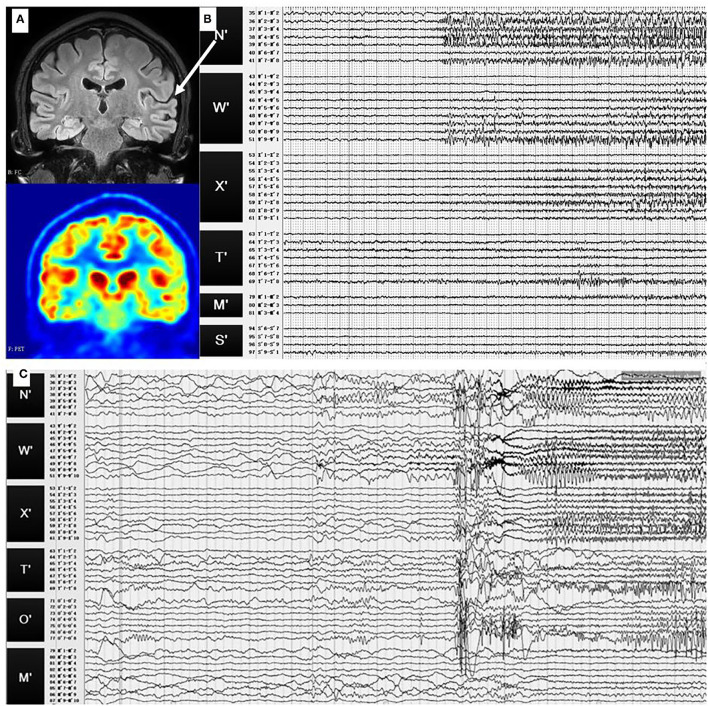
(A) Preoperative T1-weighted MRI and PET results of case 2. No abnormality was found in MRI, while hypometabolism was identified in the left superior temporal gyrus. (B) SEEG signals of the first seizure pattern. During this pattern, fast activities or sharp slow waves were identified at the left superior temporal gyrus (N′3-5), then sharp waves and spike waves spread in the left superior temporal gyrus (N′2-8, W′5-10, X′4-8). (C) SEEG signals of the second seizure pattern. There were fast activities and slow-wave oscillation at the left superior temporal gyrus (N′3-5), then the fast activities spread in the left superior temporal gyrus (W′2-9, N′2-6), and finally, spread to the other sites. White arrow: the location of the electrodes N′ in MRI (left superior temporal gyrus).
